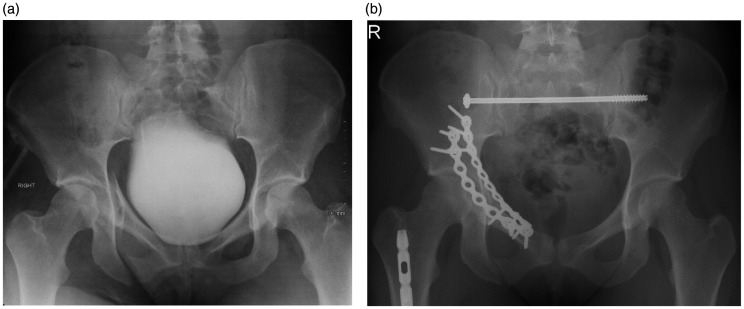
Open reduction internal fixation (ORIF). (a) Preoperative anteroposterior radiograph of a pelvic fracture with bladder contrast. (b) Postoperative radiograph of the pelvis after open reduction and internal fixation
